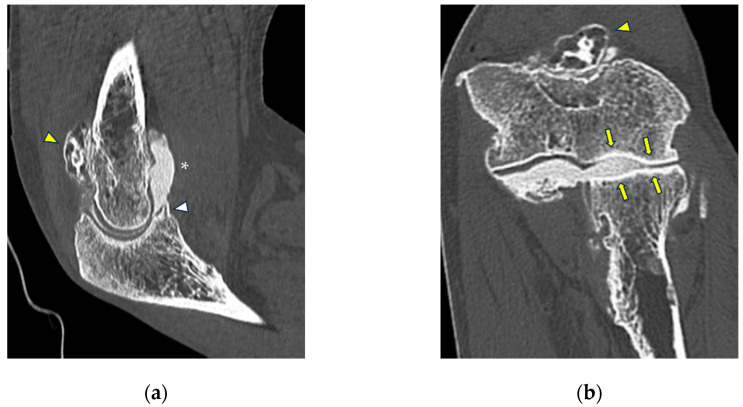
CT-arthrography of a patient with rheumatoid arthritis. (a) Sagittal image shows pathological widening of the anterior humero-ulnar recess (asterisk), osteophytosis of the coronoid process (white arrowhead) and a distended posterior recess containing multiple non-calcific loose bodies compatible with secondary synovial chondromatosis (yellow arrowheads); (b) coronal image shows abnormal morphology and complete chondral erosion of both the radial head dish and lateral humeral condyle (yellow arrows).
